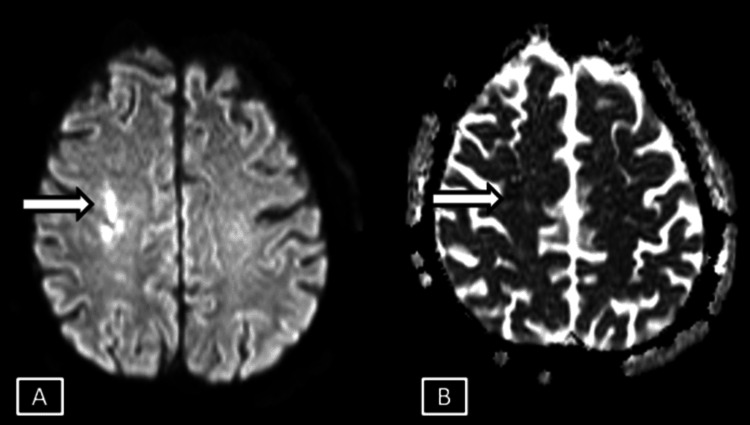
Case 3 MRI brain axial DWI (A) and ADC (B) sequences. Images show a few focal fairly defined areas of diffusion restriction with low ADC signals seen involving the deep white matter areas of right centrum semiovale represented by the white arrows. DWI: Diffusion Weighted Imaging; ADC: Apparent Diffusion Coefficient
